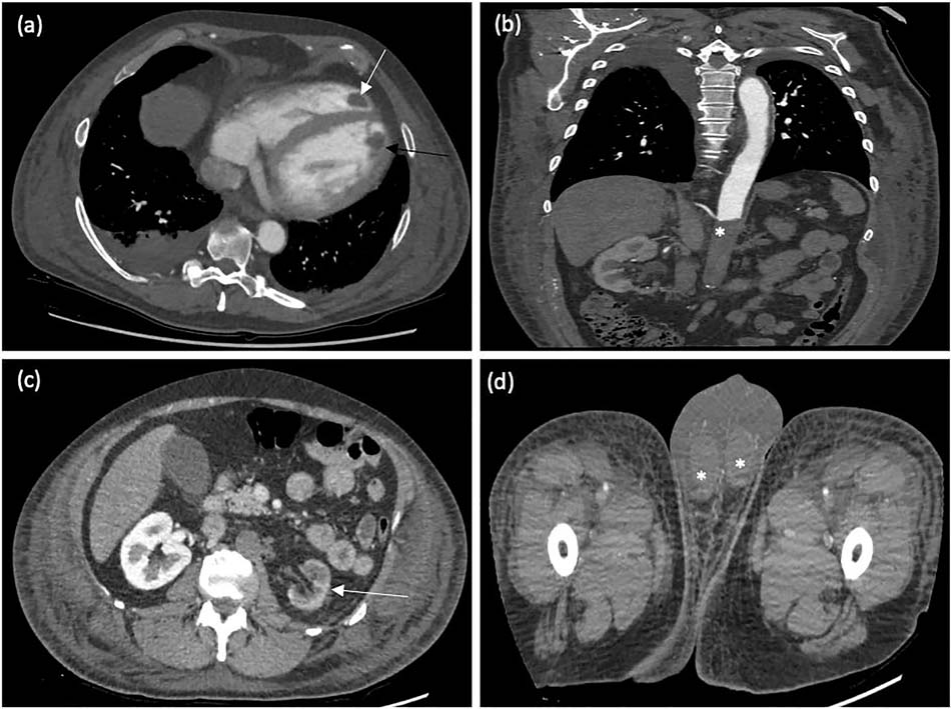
(a) Axial contrast-enhanced CT chest demonstrating biventricular apical thrombi (white arrow—RV thrombus, black arrow—left ventricular thrombus). (b) Coronal contrast-enhanced CT abdomen demonstrating extensive occlusive thrombus within the abdominal aorta (^*^). (c) Axial contrast-enhanced CT abdomen demonstrating reduced enhancement of the left kidney in keeping with infarction (Arrow). (d) Axial contrast-enhanced CT abdomen demonstrating reduced enhancement of the testes bilaterally in keeping with infarction (^*^).
